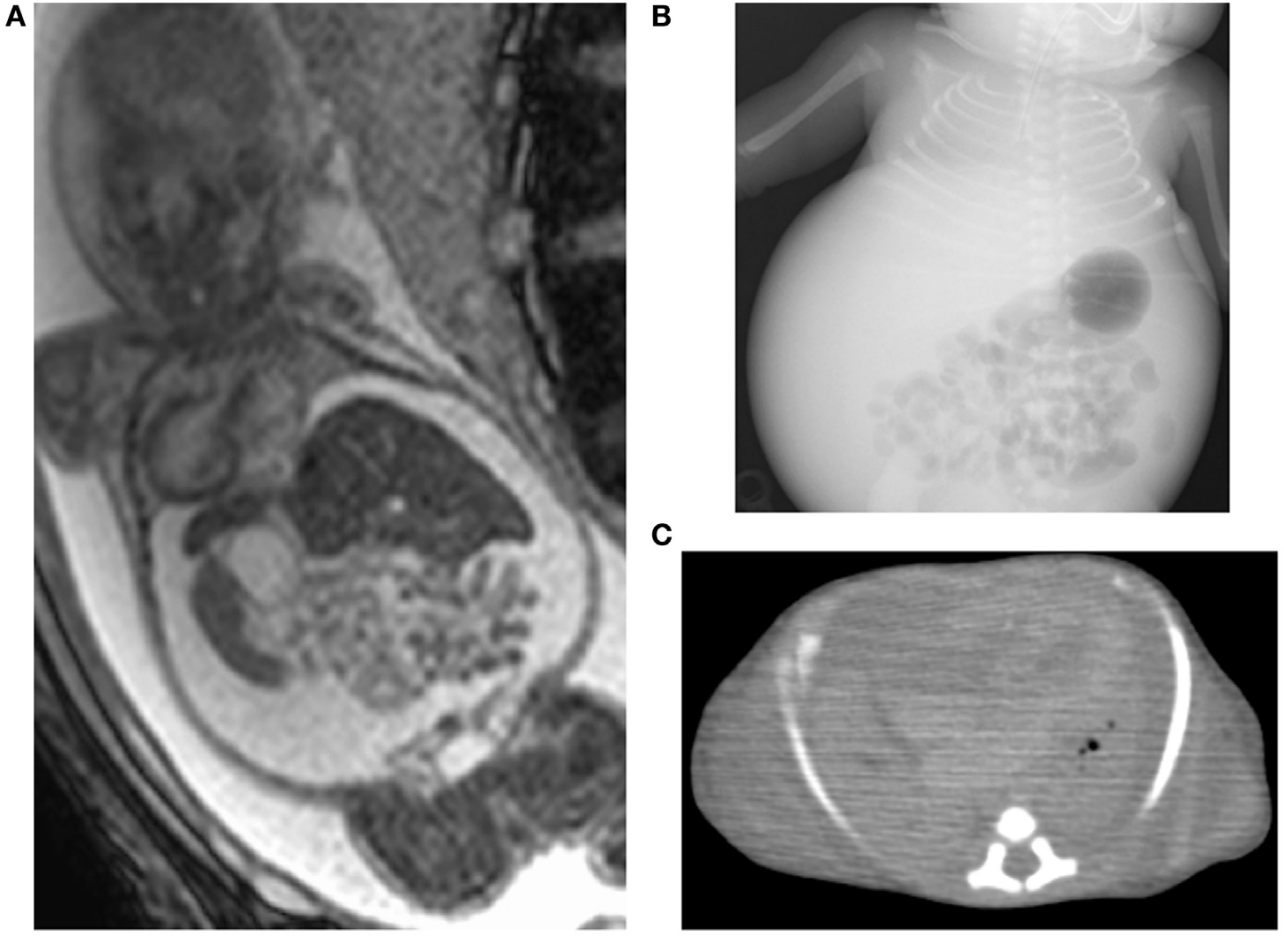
Images of case 1. Fetal magnetic resonance imaging shows massive ascites and compressed lungs (A). Chest X-ray (B) and postmortem chest CT (C) show pulmonary hypoplasia.
